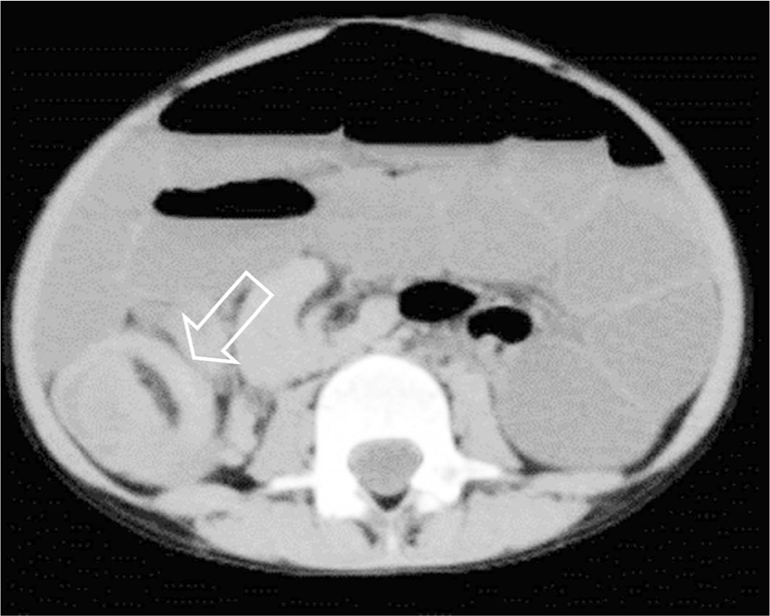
Axial postmortem CT in a boy age 4 years 4 months shows an ileocolic intussusception (*arrow*). At autopsy a Meckel diverticulum was found as a pathological lead point
